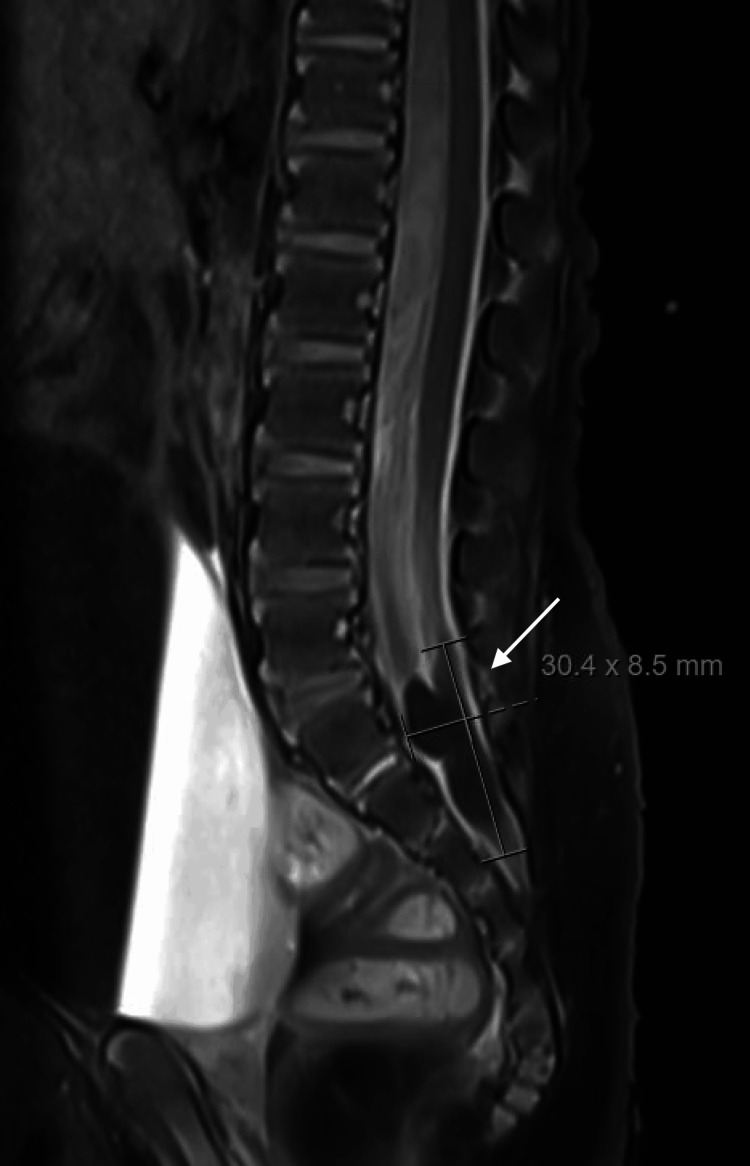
T1-weighted turbo spin-echo fat suppression spinal MRI (sagittal view) demonstrating an intradural cyst at the L5–S4 levels (white arrow) The intradural cyst was only detected on a dedicated spine MRI, highlighting the need for specific imaging to identify this anatomic anomaly.
